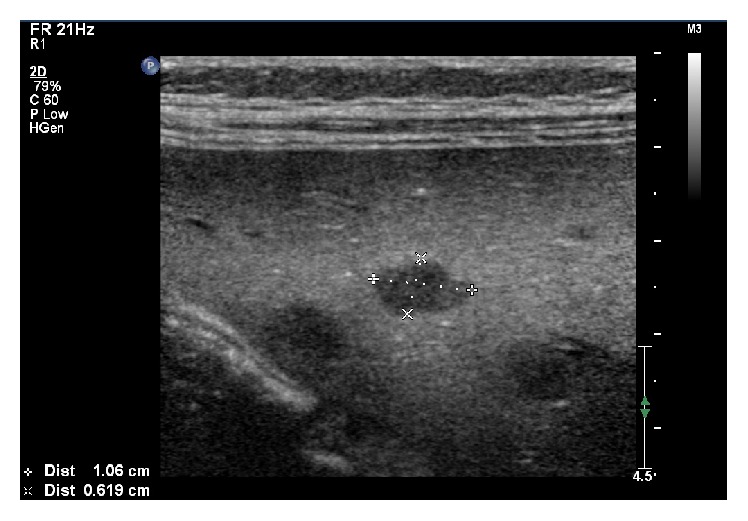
Fungal microabscesses in hepatic ultrasonography at diagnosis.
